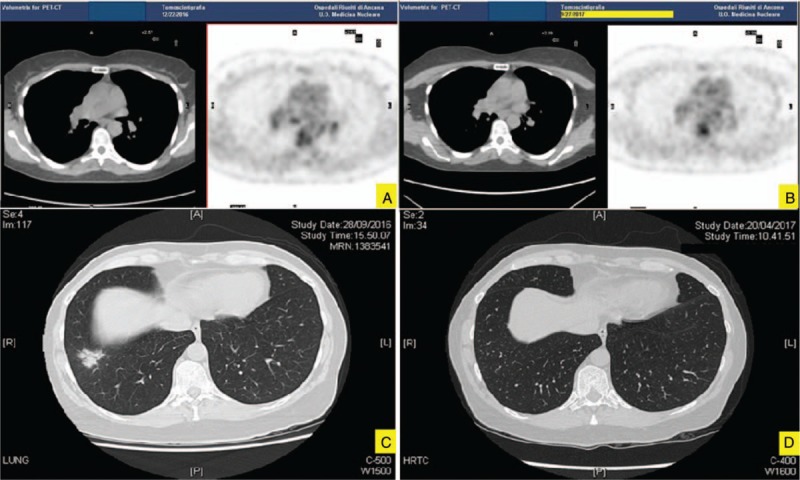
Positron emission tomography (18FDG PET-CT) and HRCT images before (A, C) and after (B, D) a single course of rituximab: attenuation of 18-FDG uptake in lymph nodes and resolution of the nodular lesion at the inferior right pulmonary lobe at 12 months follow-up.
